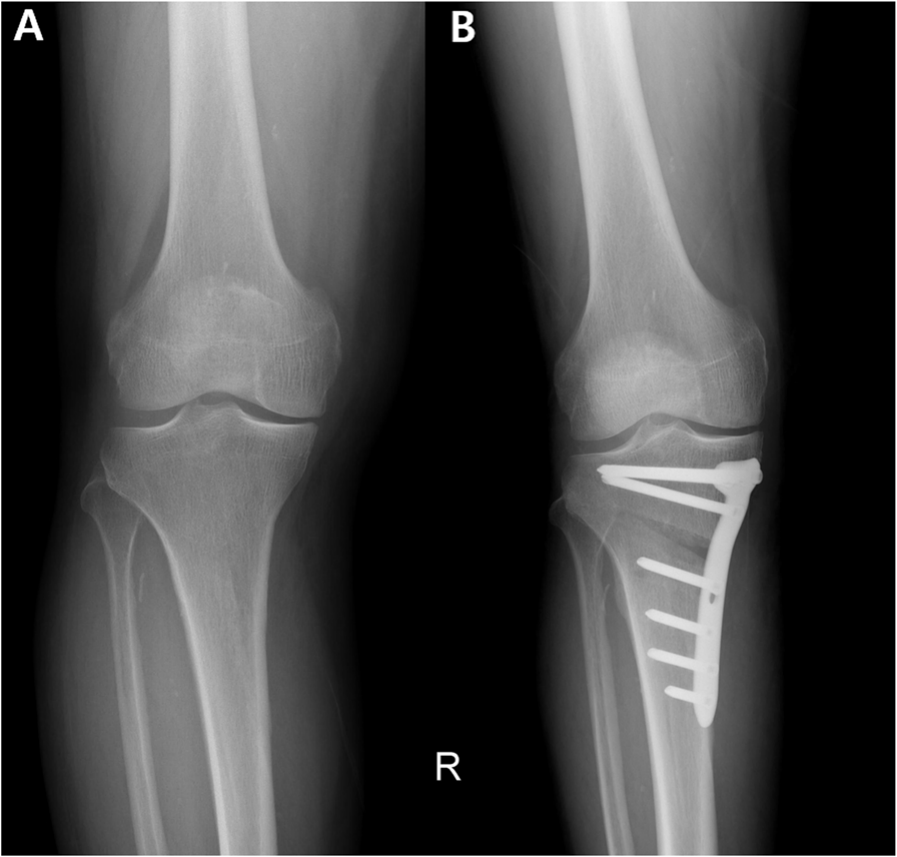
Preoperative (A) and postoperative (B) radiographs of medial opening wedge high tibial osteotomy
